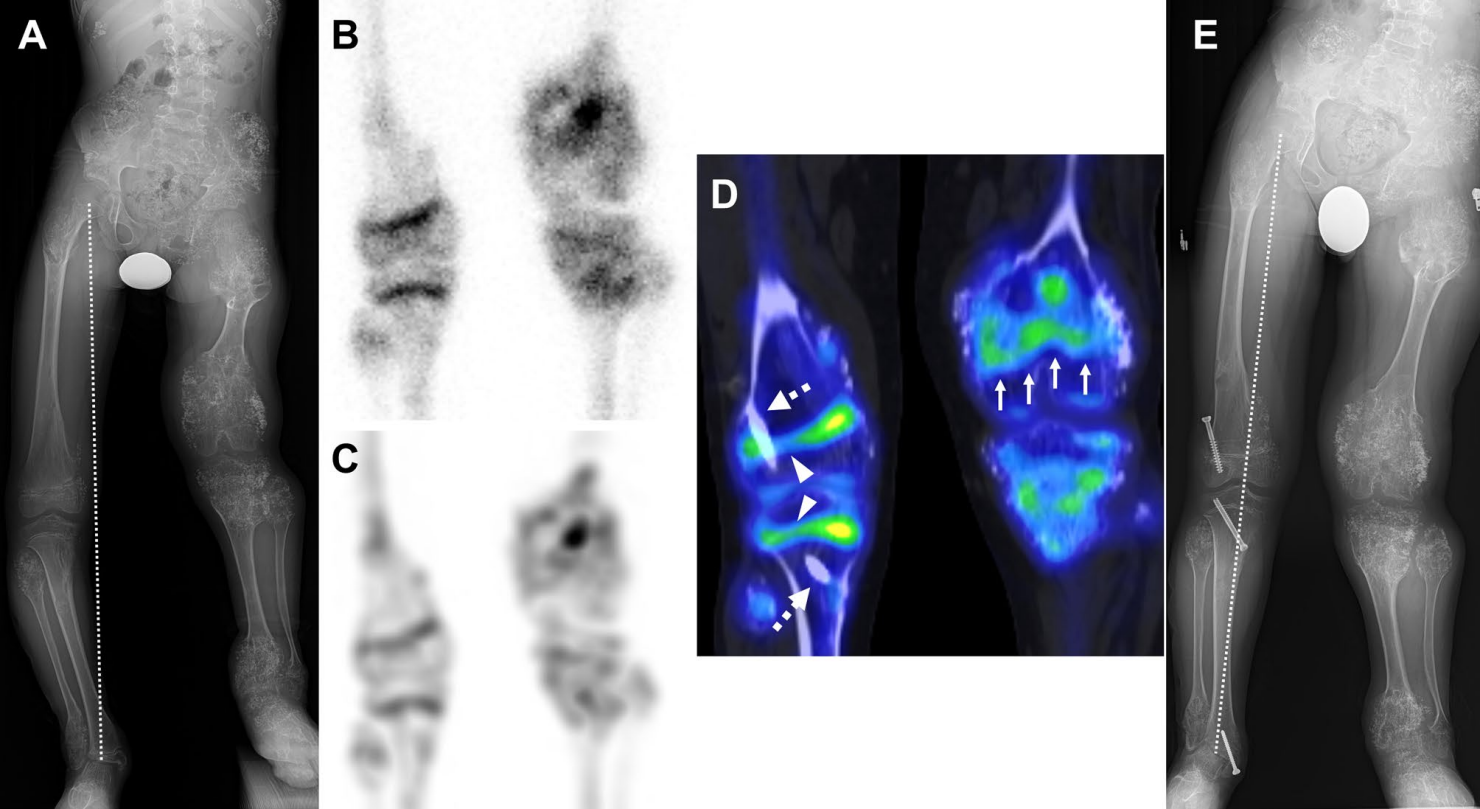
Advantage of bone SPECT/CT for evaluating physes whose locations are unclear on conventional radiographs. A 9.6-year-old boy with metaphyseal chondromatosis with D-2-hydroxyglutaric aciduria exhibited shortening and genu valgum on the left side and genu varum on the right side (A). The locations of the physes of the left leg are vague on conventional radiography (A). It is difficult to evaluate physeal activity of the left leg on planar scintigraphy (B) and SPECT (C) because uptake of ^99m^Tc-DPD can occur both in the tumor and physis, and the physis may be deformed. The uptake of the radiotracer by the tumor and physis can be differentiated on fused SPECT/CT, owing to its high resolution and anatomic information (D). Physeal activity in the left distal femur (arrows) is identified despite a decrease (D). Screws inserted for temporary hemiepiphysiodesis (dashed arrows) have resulted in hypoactive lateral physes (arrowheads) compared to the normal activity of the medial physis of the right knee (D). The physeal activity of the right knee indicates that genu varum of the right leg will improve (A and E)
